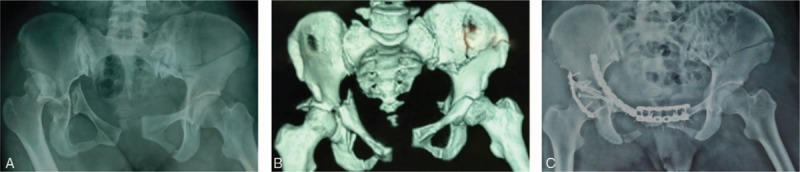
(A) This photo indicated fractures of left iliac wing, bilateral acetabulum, double ischial branch, right inferior pubic branch, and sacrum wing. The left iliac wing fracture involved the left iliac joint along with separations of right hip joint, symphysis pubis and bilateral sacral iliac joint. (B) It is the 3-dimensional CT reconstruction of broken pelvic. (C) Fractures of right acetabulum and superior ramus of pubis were fixed with porous plates and screws.
